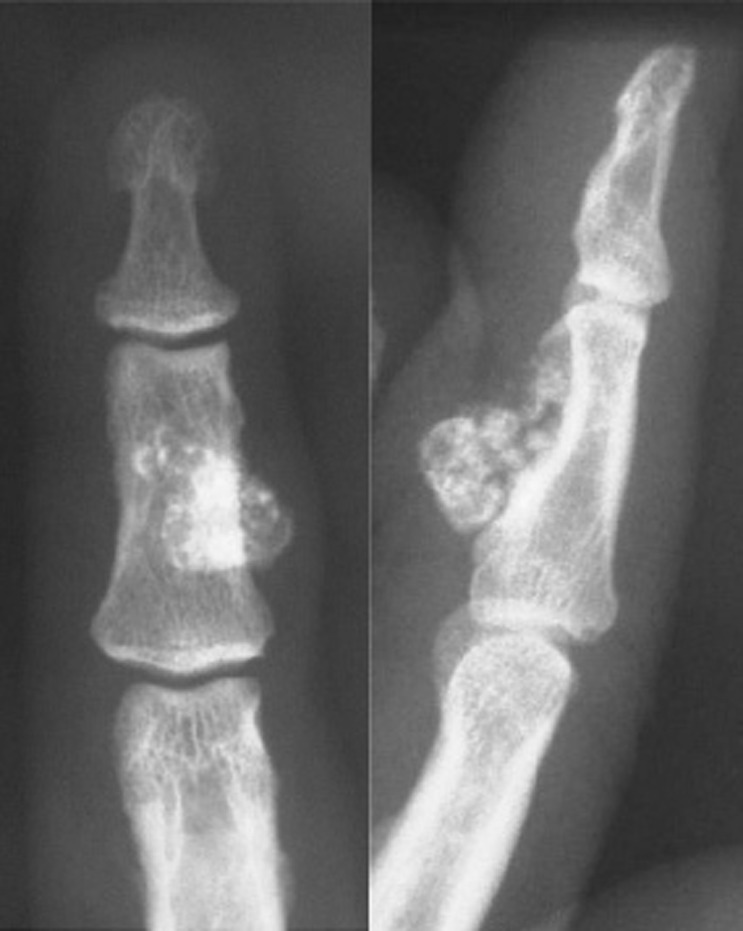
A 52-year-old man presenting with a 5-year history of mass on the palmar aspect of the right index finger. Anteroposterior and lateral radiographs of the right index show a juxta-cortical calcified lesion of the middle phalanx with cortical erosion.
